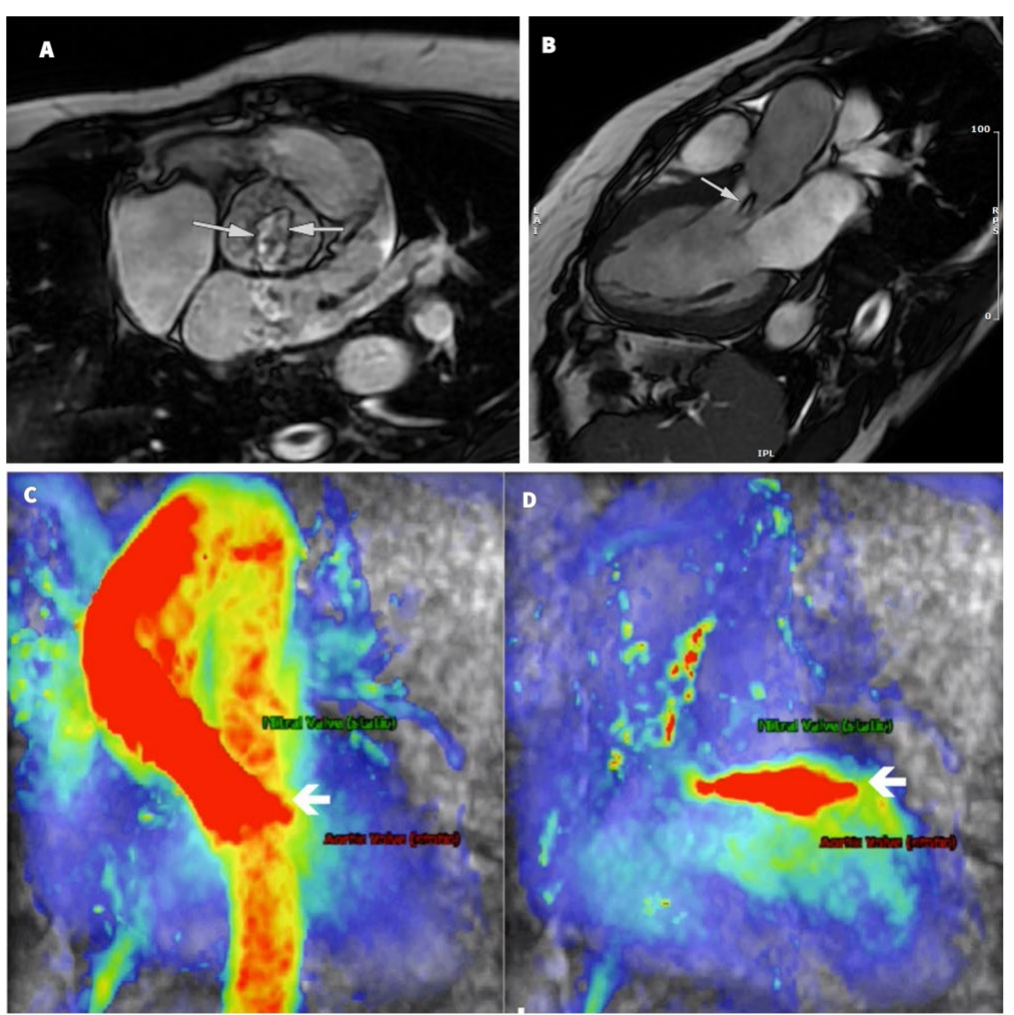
Cardiac magnetic resonance imaging: (A) Cine Fiesta sequence in the transverse plane: bicuspid aortic valve type 0, lateral (arrows). (B) Cine Fiesta sequence 3: aortic insufficiency jet (arrow). (C,D) Sequence 4D flow: aorta in systole (C), blood flow coded in red (arrow), and in diastole (D), highlighting a jet of severe aortic valvular insufficiency with oblique orientation (arrow).
